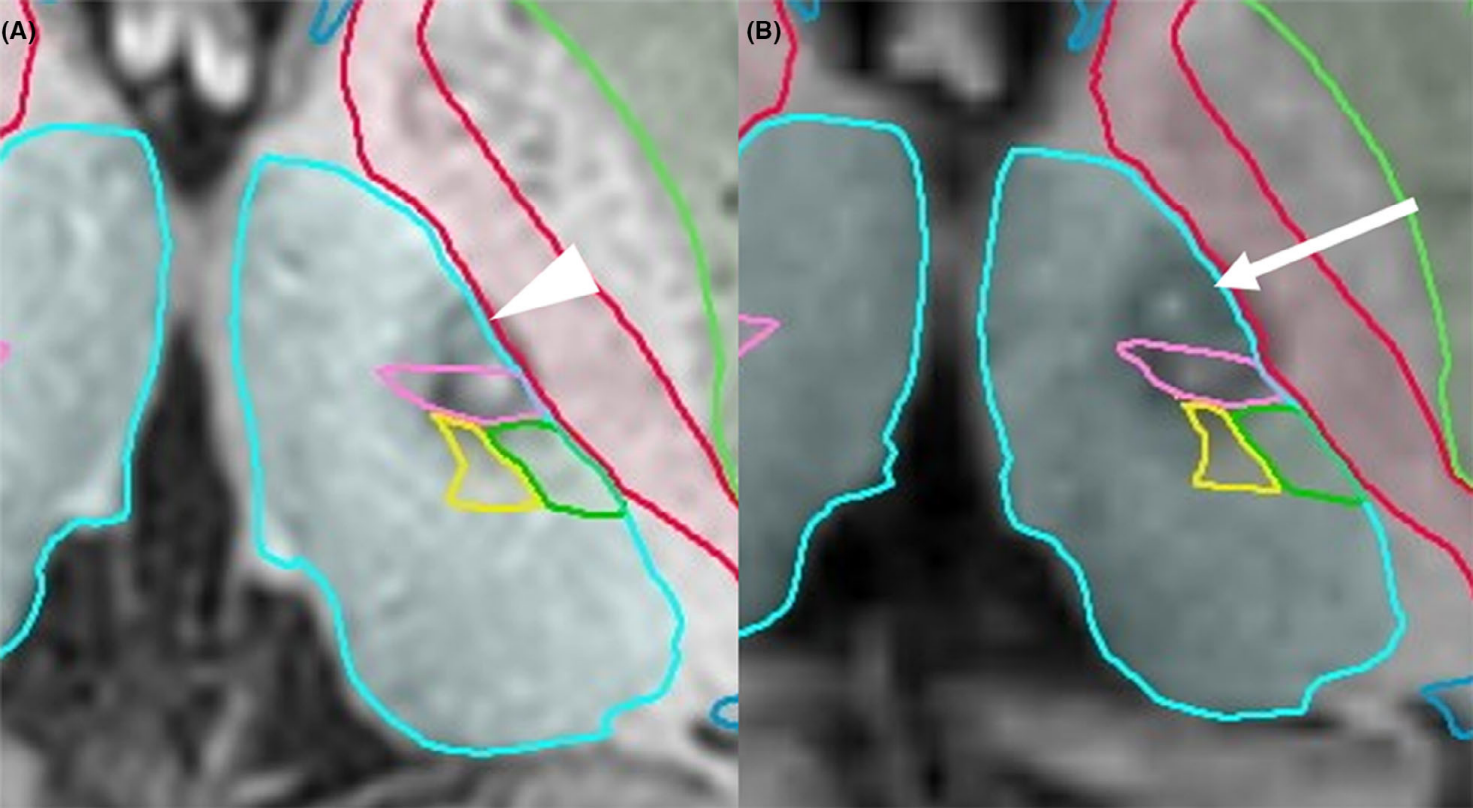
Postoperative MRI images in a patient with focal hand dystonia developed after thalamotomy. Task‐specific tremor was well controlled by the first thalamotomy (A). Four months after the first thalamotomy, focal hand dystonia developed. The second thalamotomy resulted in complete resolution of the focal hand dystonia (B). The Vop lesion in the first thalamotomy (arrowhead) is considerably smaller than that in the second thalamotomy (arrow). MRI, magnetic resonance imaging.
